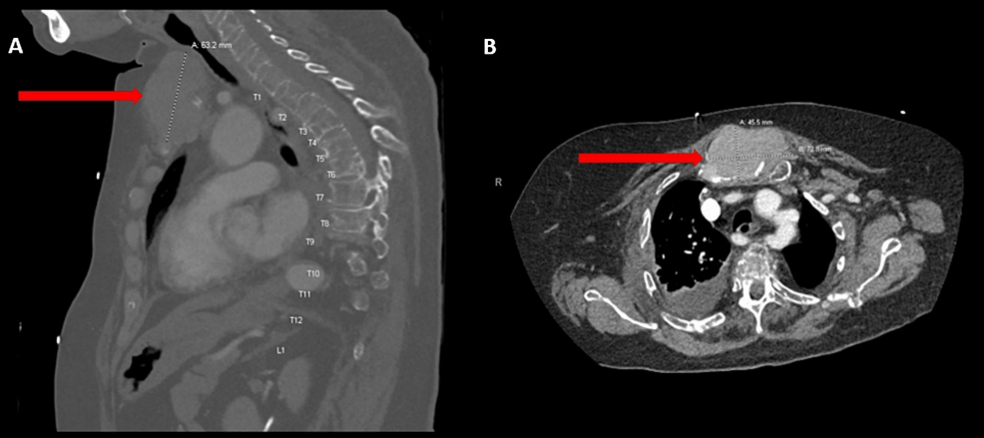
Large extensive soft tissue mass measuring 4.5 × 7.3 × 6.3 cm centered about the sternum on CTA; A: sagittal view, B: axial view CTA, computed tomography angiography
